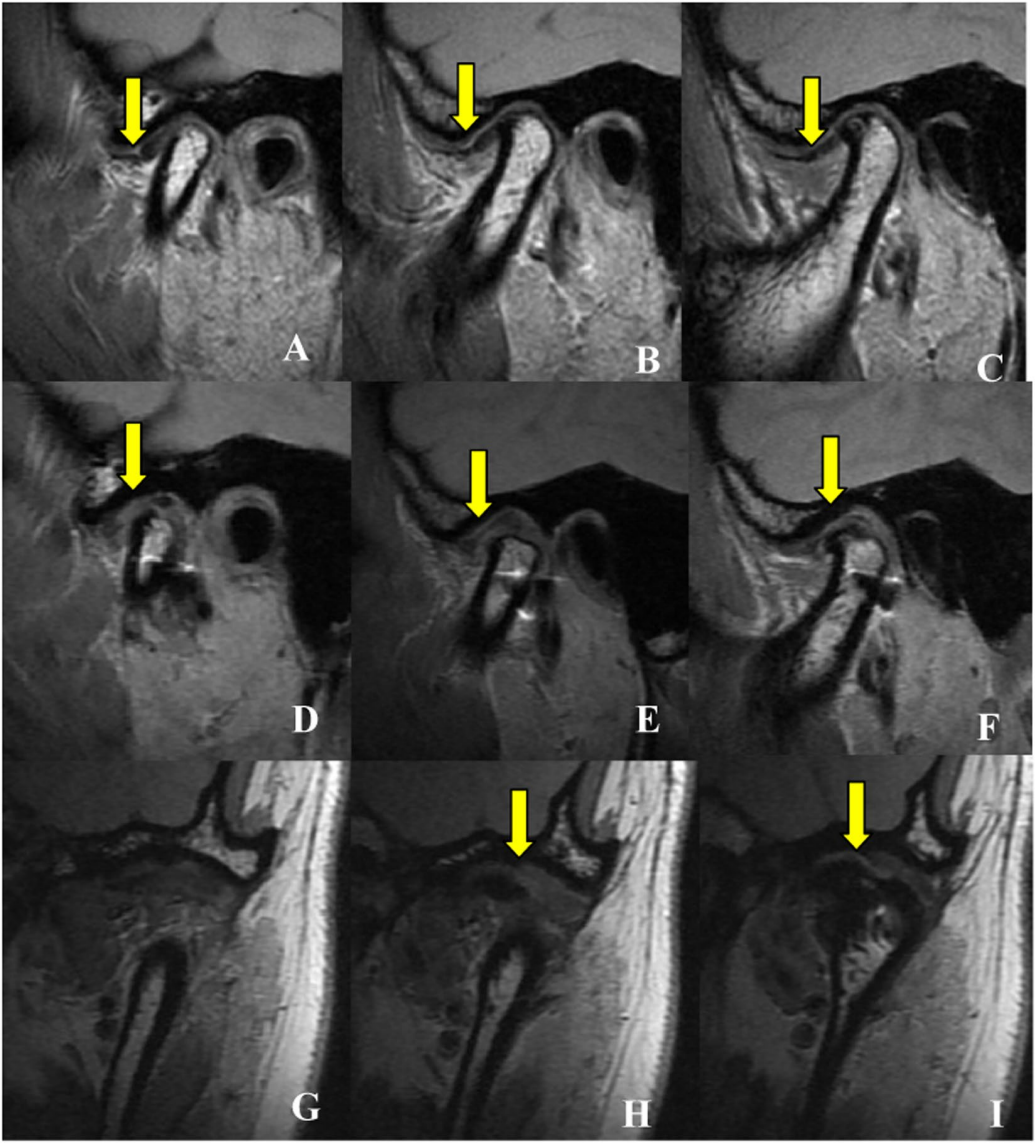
Female patient aged 26 years, with internal derangement stage IV right side and stage V left side, disc position on MRI before and after surgery to left side. Internal (A), middle (B) and lateral (C) layers of the long axis of the condylar process before surgery, in sagittal plane. Images demonstrate that the disc is anteriorly displaced in the closed-mouth position and the disc is thin with a potentially broken area (yellow arrow). Internal (D), middle (E) and lateral (F) layers of the long axis of the condylar process after surgery, in sagittal plane. Images demonstrate that the displaced disc is repositioned to the normal position but the disc image appears broken (yellow arrow). Middle (H) and posterior (I) layers of the long axis of the condylar process, after surgery, in coronal plane. The images demonstrate that the displaced disc is repositioned to the normal position but the disc image appears broken (yellow arrow), and in the anterior (G) layer the displaced disc is not seen.
